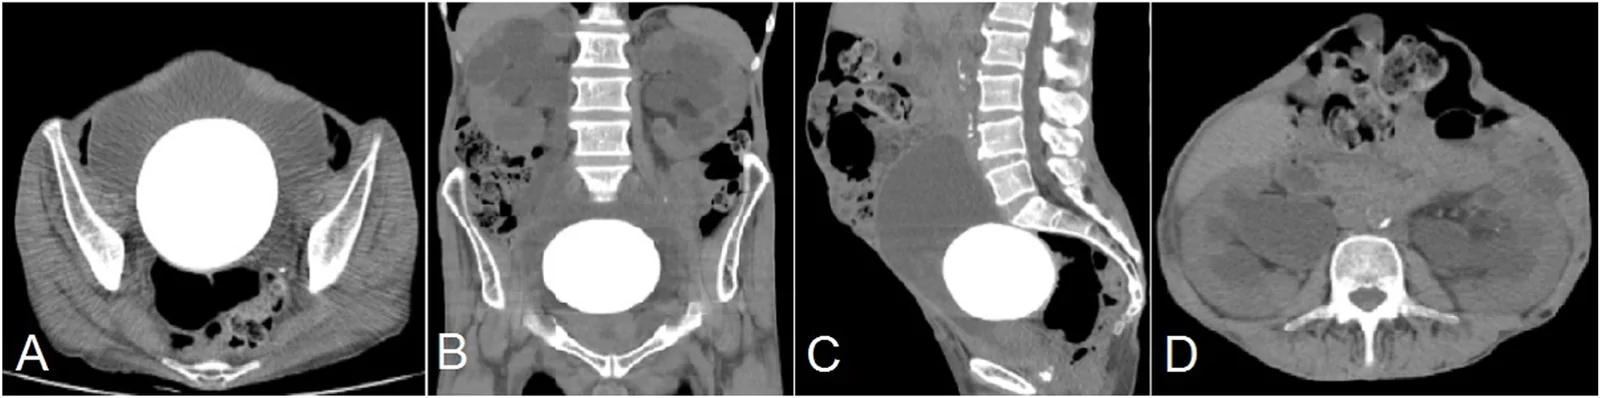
Computed tomography scan. Axial (A), coronal (B), and sagittal (C) views of the bladder, and axial view of the kidneys (D), demonstrating a large bladder stone and severe bilateral hydroureteronephrosis.
